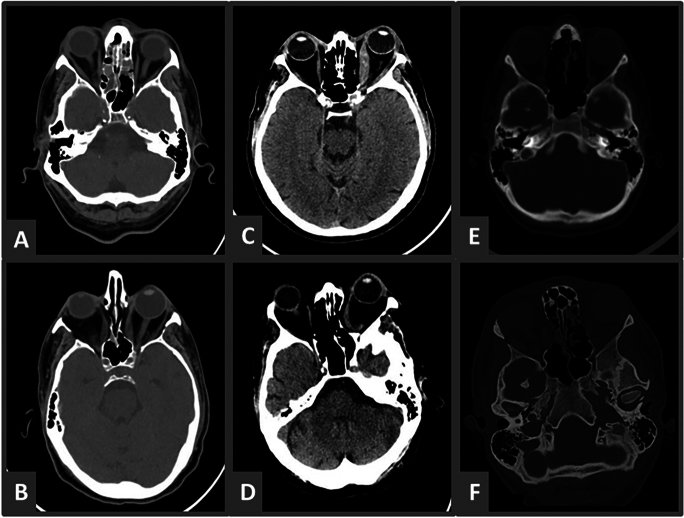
Pre and post-operative axial CT scans. A, B Patients 17 (55M, BE medial and lateral walls). C, D Patient 2 (61M, LE medial wall only). E, F Patient 27 (18F, RE, Medial and lateral walls)
